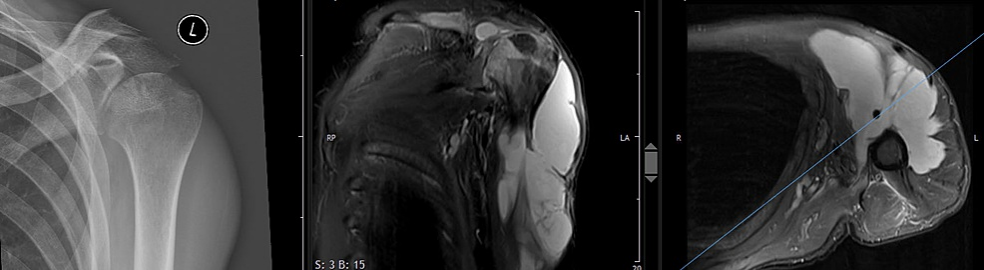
Anteroposterior radiograph (left), fat-saturated proton density-weighted coronal (middle), and axial MR image (right) of the left shoulder made 12 days after the second intraarticular cortisone infiltration A huge liquid accumulation under the thinned-out deltoid muscle, extending under the supraspinatus muscle and far distally into the upper arm, is visible. The humeral head structure is altered and the articular cartilage has disappeared.
